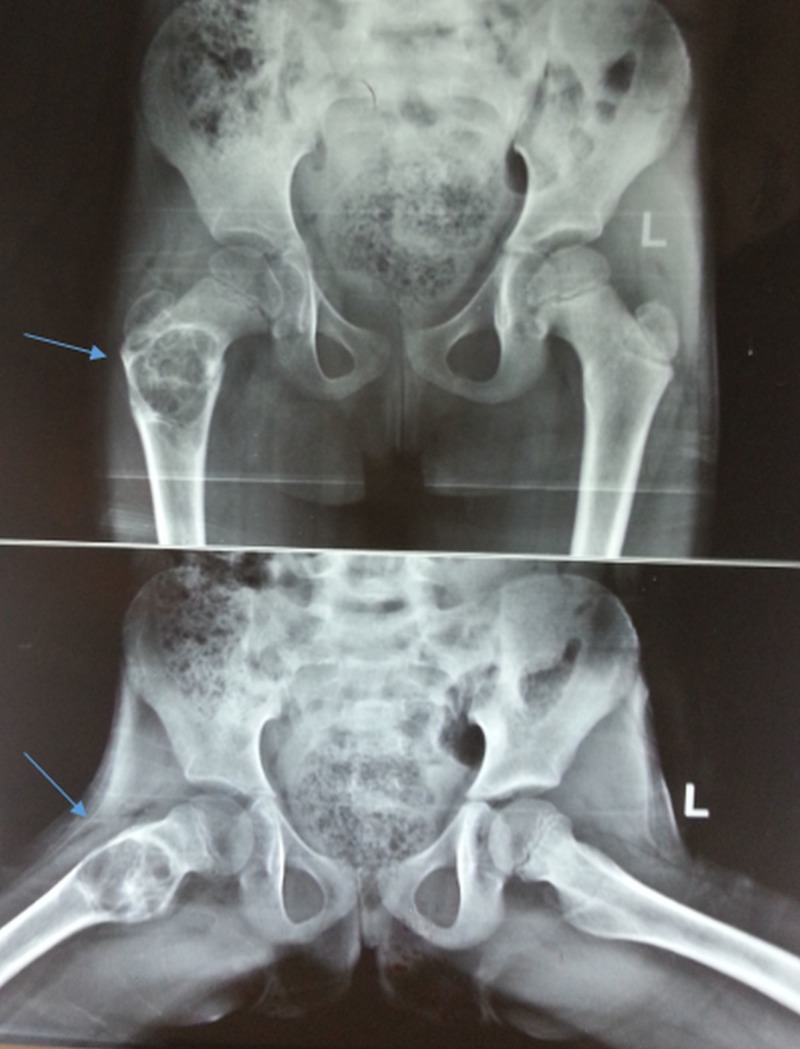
Preoperative x-ray (anteroposterior and lateral) Plain radiographs show lytic lesion in proximal portion of the right femur
